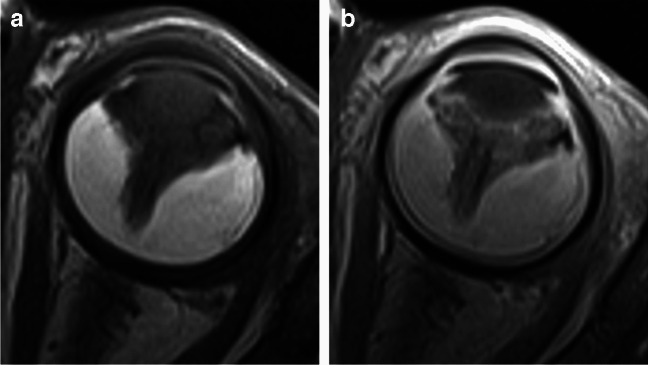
Axial T1-weighted images of the globe without (a) and with contrast agent (b) show Y-shaped retinal detachment with prominent hyperintensity of the exudate and enhancement of the cone-shaped retrolental tissue in this patient with PHV
